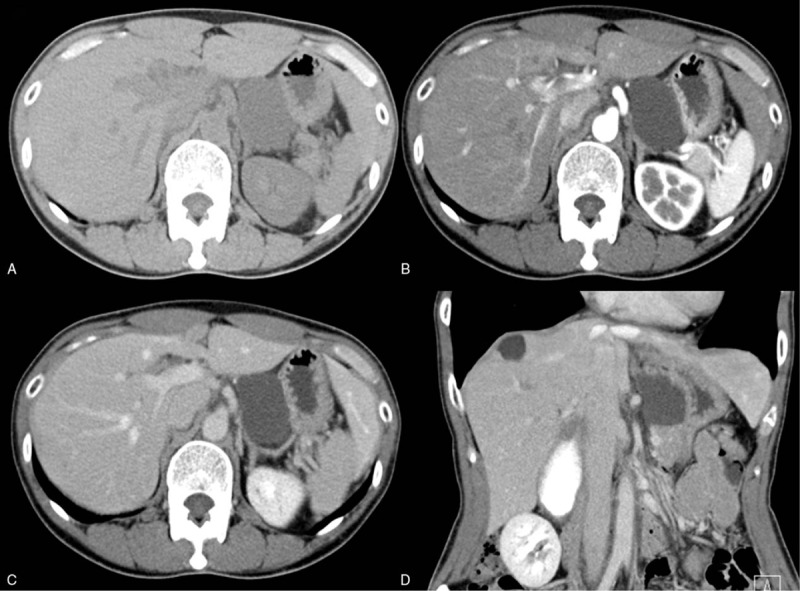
Multiphasic contrast-enhanced computed tomography findings. Unilocular cystic lesion in lesser curvature of stomach has no separation or mural nodules, and a thin wall without contrast enhancement. (A) Axial plain CT image, (B) Arterial phase and (C) delayed phase of the dynamic contrast study, and (D) Coronal CT image. CT = computed tomography.
